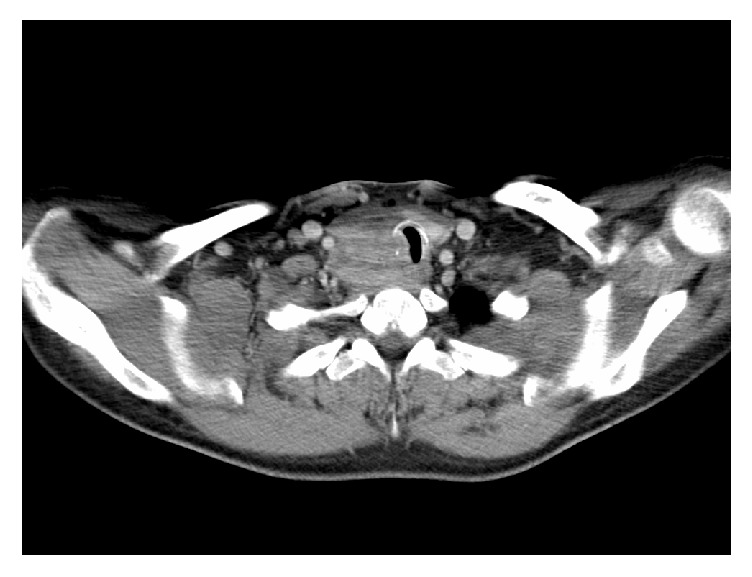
Neck CT scan showing the invasion of the trachea.
